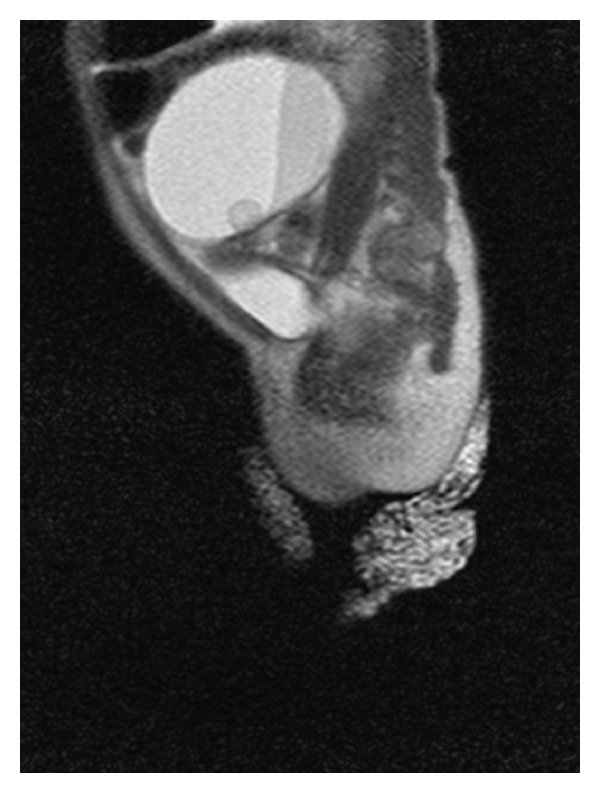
A haste sagittal MBH MRI image demonstrates a 4.5 × 5.6 × 4.5 cm cystic mass with layering hemorrhagic or proteinaceous elements within it. A nodular internal 1.0 cm cystic portion against the inner wall of the large mass is also seen.
